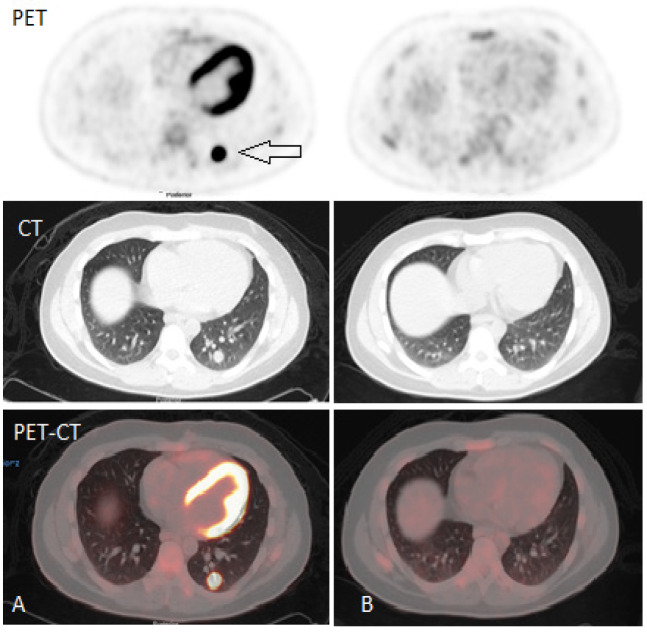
23-Years Old Male with Testicular Cancer. Right sided orchidectomy done one year back. CT scan showed solitary lung nodule on the left side. Further characterization was requested through FDG PET-CT scan (injected dose=4.7mCi) which showed hyper metabolic pulmonary nodule (A) suggestive of metastatic disease relapse. B) post therapy image show complete resolution
